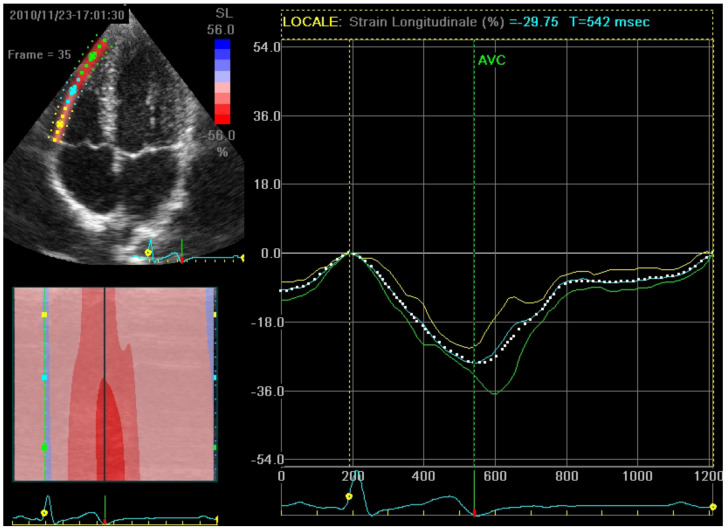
Top left: a RV free-wall in a 4-chambers view divided into three segments by the STE software, each providing its own longitudinal strain value. Right: the curve of each segment’s longitudinal strain and the average curve representing RVFWLS. RV: right ventricle; STE: speckle tracking echocardiography, RVFWLS: right ventricle free-wall longitudinal strain.
